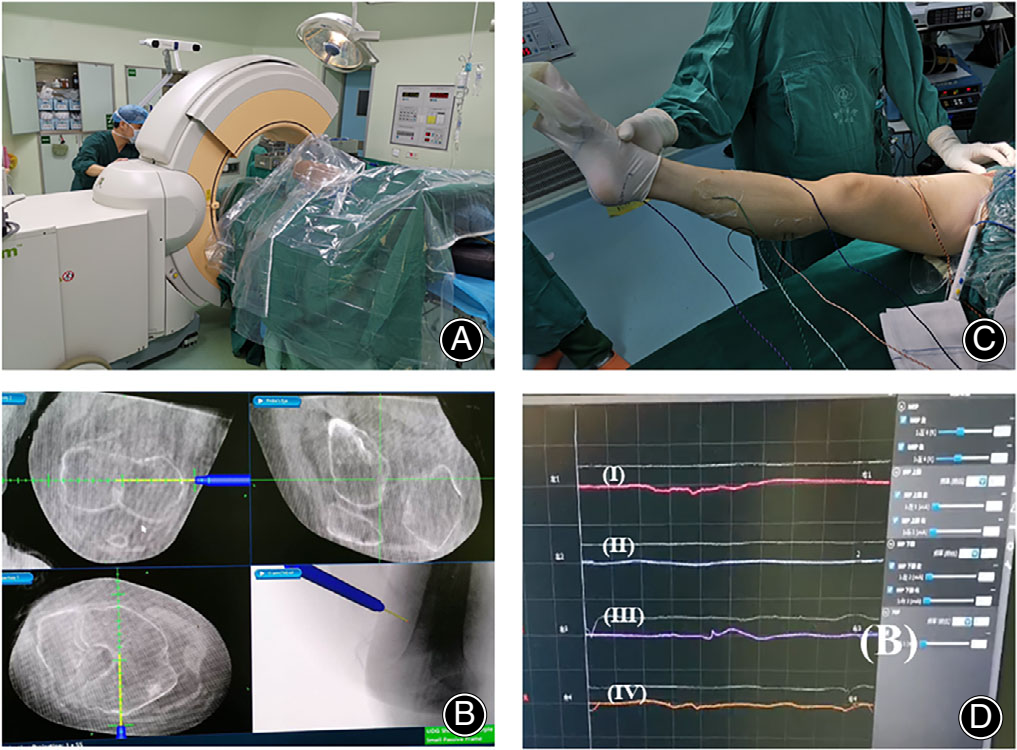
Application of special intraoperative instruments to increase accuracy. (A) Intraoperative CT scanning and navigation are beneficial to precisely determine the degree of femoral anteversion. (B) The transepicondylar line of the femoral condyle is used as a reference, and the femoral anteversion is set to 10º–15°. (C) Introduction of intraoperative nerve monitoring in THA *via* DAA in Crowe type III‐IV DDH. The electrodes are placed into key muscles percutaneously to detect the nerve status during hip reduction. (D) An abnormally evoked potential strongly indicates overlengthening and potential damage to the nerve.
